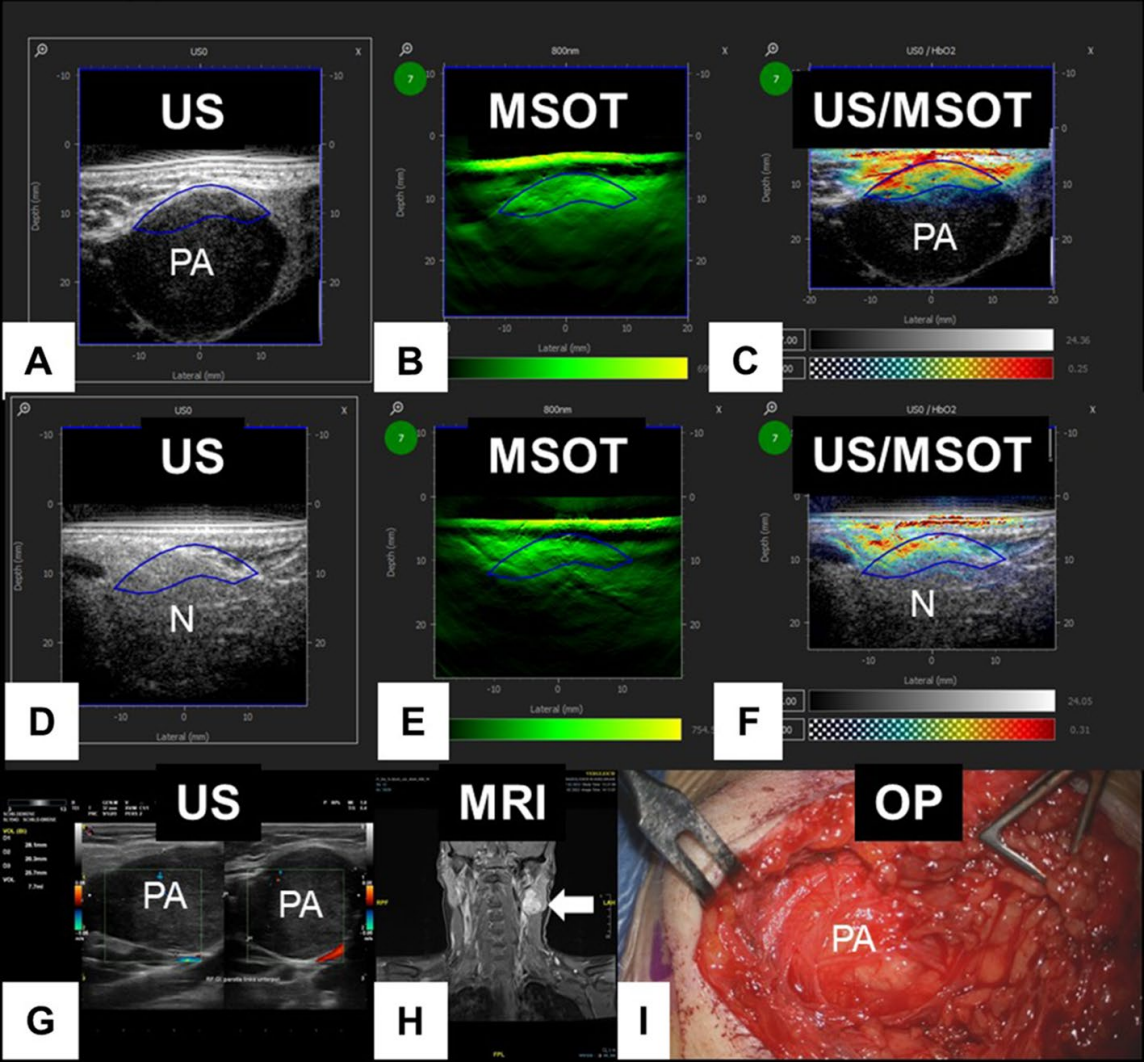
Example of a pleomorphic adenoma (PA) of the left parotid gland. (A–C) Parotid gland with tumor; (D–F) contralateral healthy parotid gland. (A) Ultrasound (US) image of the MSOT system; PA circled in blue. (B) MSOT image of 800 nm; Region of interest (ROI) circled in blue. (C) Hybrid US/MSOT image; ROI circled in blue. (D) US image of the MSOT system; ROI in normal (N) salivary gland tissue circled in blue. (E) MSOT image of 800 nm; ROI in normal tissue circled in blue. (F) Hybrid US/MSOT image; ROI in normal tissue circled in blue. (G) High-resolution image with normal US device. (H) Coronal magnetic resonance imaging (MRI) of the neck showing the tumor (arrow) in the parotid gland. (I) Intraoperative (OP) situs. The tumor is exposed in the parotid gland.
